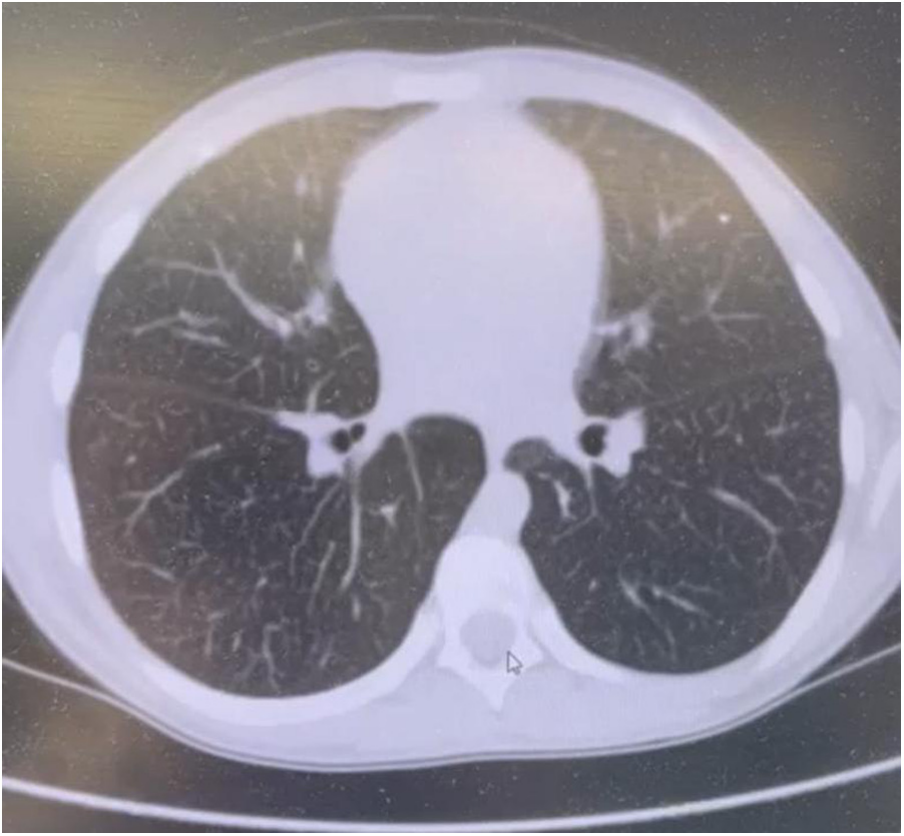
Chest CT scan of a patient with acid sphingomyelinase deficiency (ASMD) type A/B disease.
